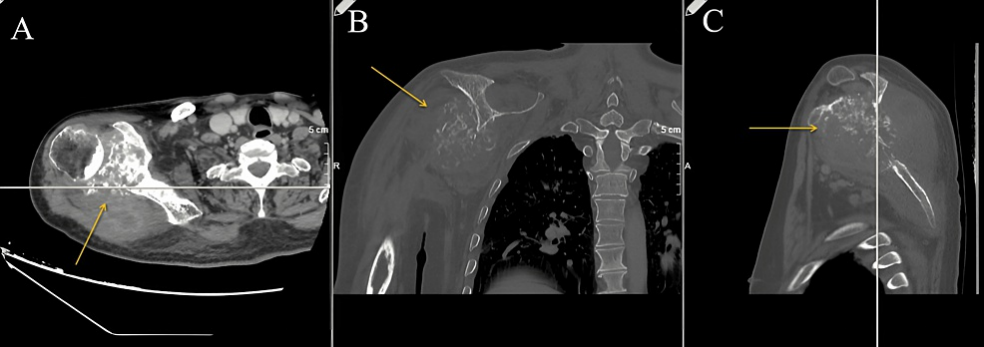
Preoperative axial (A), coronal (B), and sagittal (C) CT images of the shoulder. Lytic infiltrative lesions were observed extending from the scapula corpus to the glenoid and coracoid process and completely infiltrating the glenoid and sclerotic lesions in the part of the humerus head facing the glenoid
